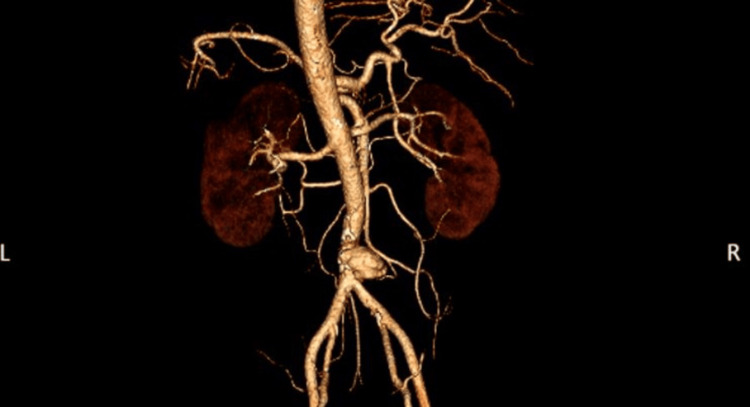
CT angiography of the abdomen showing a saccular aneurysm over the distal infrarenal abdominal aorta, measuring approximately 2.1 x 2.1 x 2.0 cm
